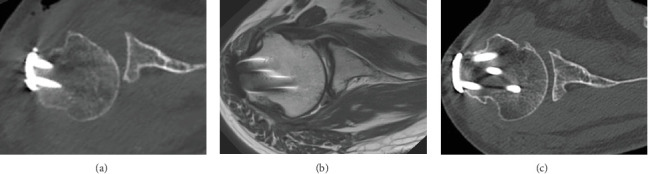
Postoperative CT and MRI examinations. (a) CT on postoperative Day 1. (b) MRI 3 months postoperatively. (c) CT images taken 12 months postoperatively. The centric position of the humeral head showing gradual improvement. CT: computed tomography; MRI: magnetic resonance imaging.
